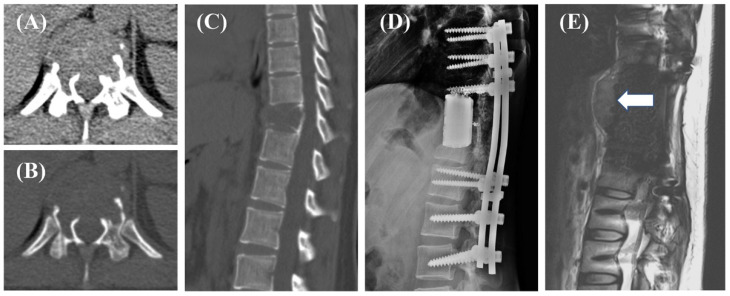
A 29-year-old female, axial (A,B) and sagittal (C) CT images showed the bone destruction of the T12, managed with total en bloc spondylectomy (TES, (D)). Postoperative pathology suggested a high expression of RANKL in the tumor. The patient did not undergo radiotherapy. At a 10-month follow-up review, recurrence was detected ((E), arrow).
